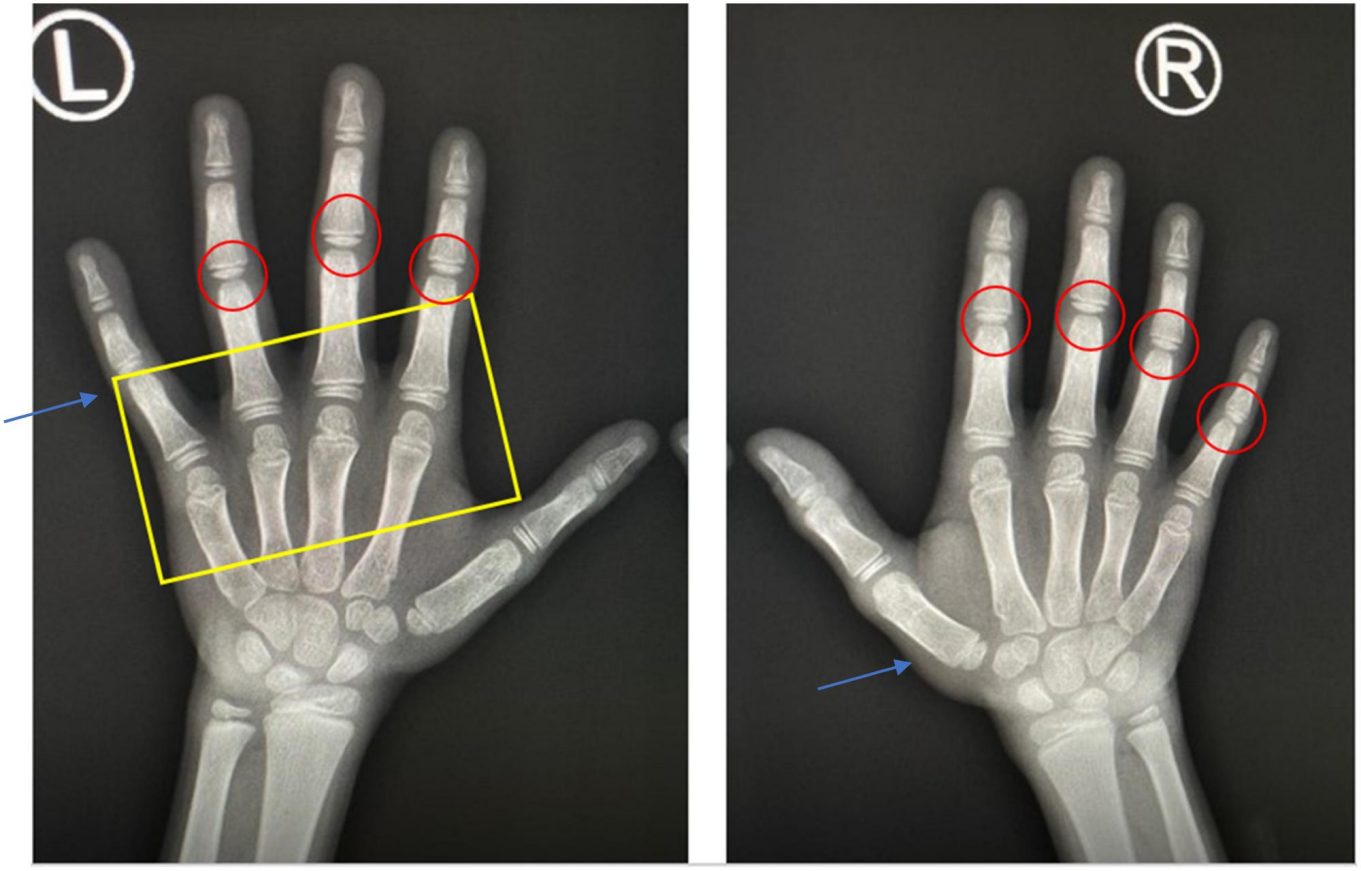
Initial bilateral hand radiographs of the patient. The red circles highlight joint space narrowing in the PIP joints bilaterally, while the yellow rectangle outlines areas of periarticular osteopenia, more prominent in the left MCP region. These findings, observed in the absence of overt joint swelling or effusion, are consistent with early radiologic features of DS
